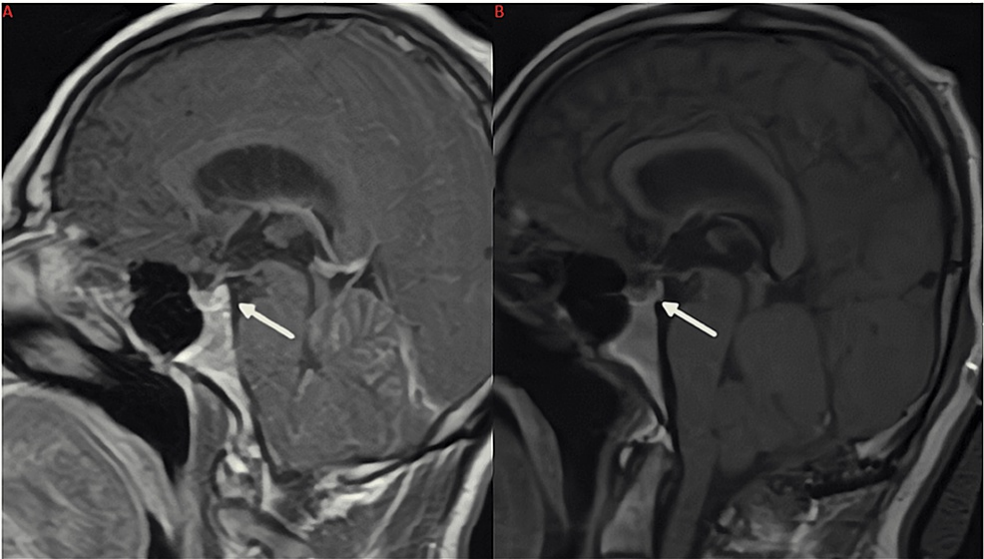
(A) Preoperative MRI (left) is shown for comparison. (B) MRI of the brain postcontrast in the sagittal plane A partially empty sella sign is seen on the postoperative MRI that was not present prior to surgery (yellow arrows pointing to sella). The empty sella sign is a radiographic indicator of hydrocephalus MRI: magnetic resonance imaging
